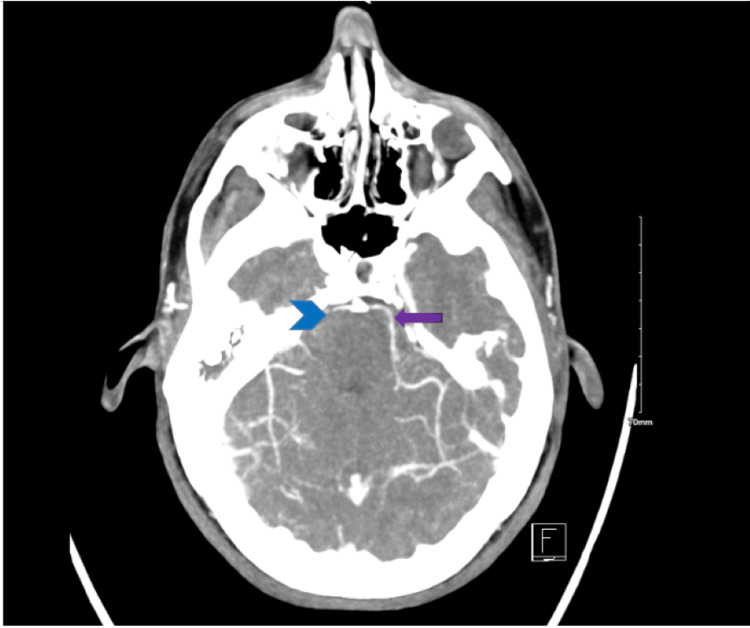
CTA of the head and neck The blue arrowhead shows the loss of filling defect of the right PCA while the purple arrow indicates continued flow of contrast in the left PCA CTA: computed tomography angiography; PCA: posterior cerebral artery
